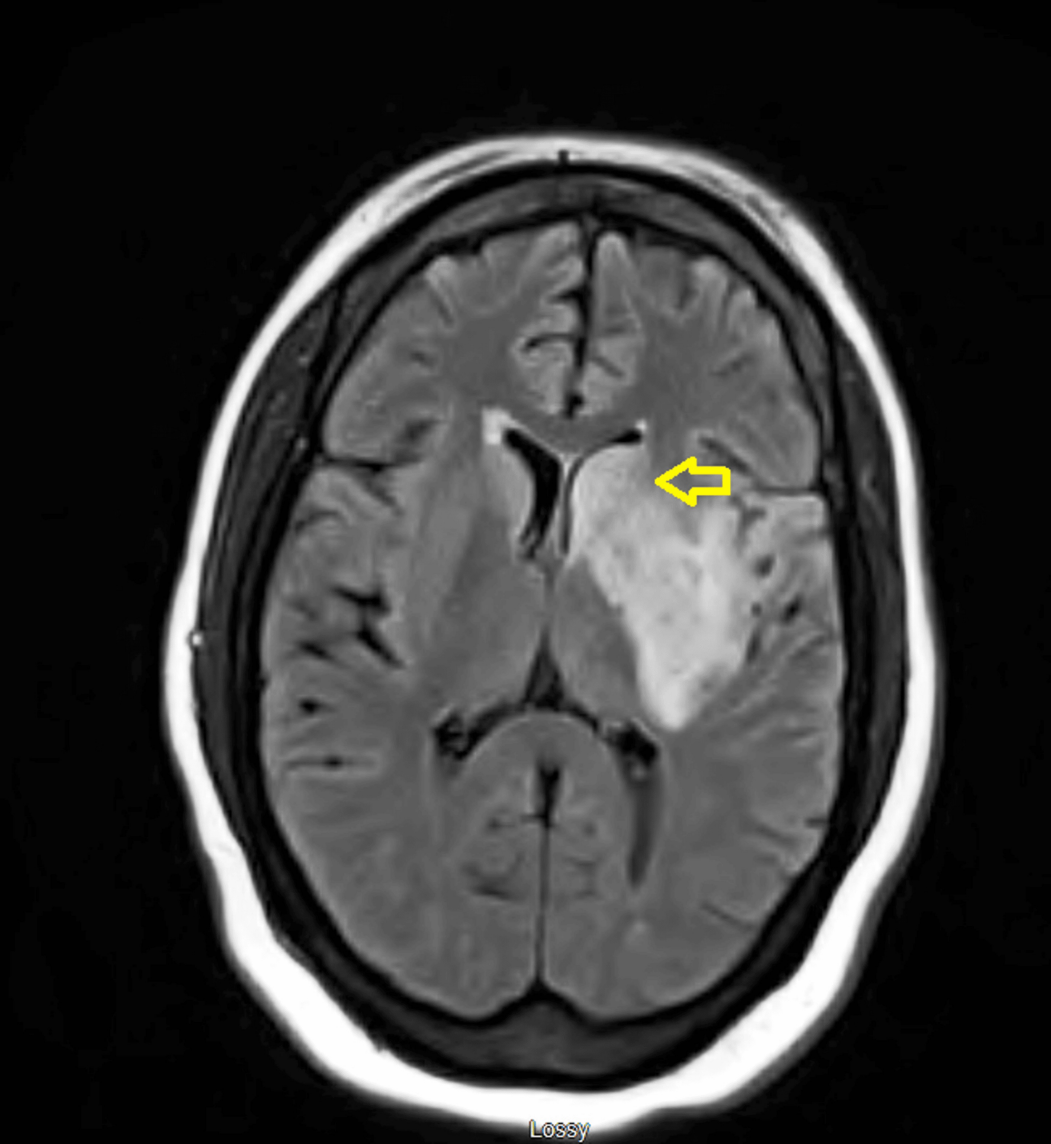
MRI showing infarction in the left insula and basal ganglia region with mass effect and midline shift to right.
